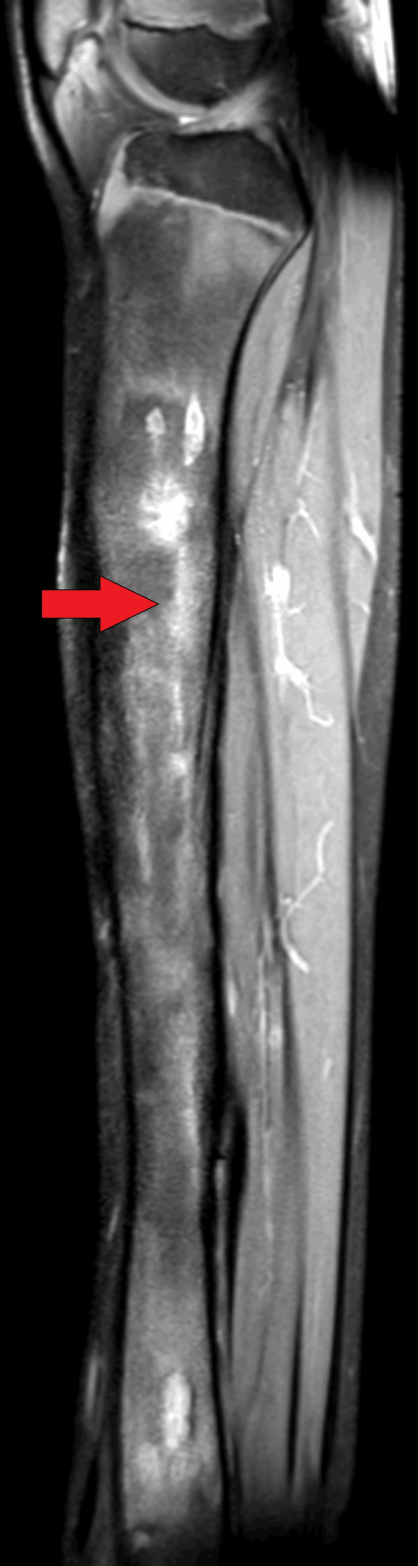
Sagittal PD-Fat-Sat MRI showing extensive hyperintensity involving the marrow of the diaphysis of tibia suggestive of marrow edema PD-Fat-Sat: proton density fat suppression image
